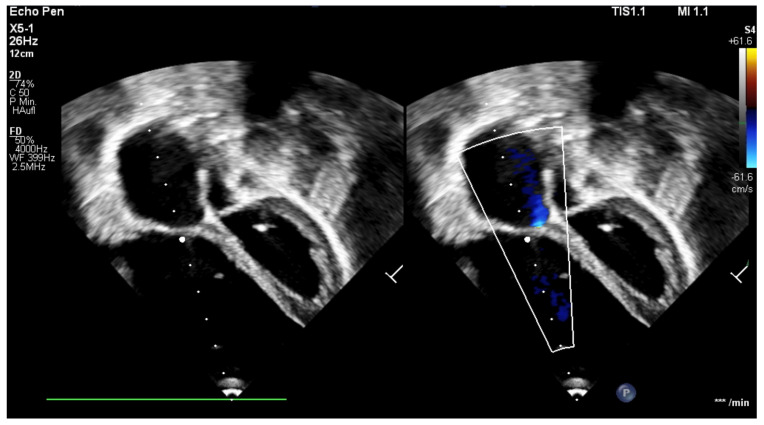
Echocardiography, four-chamber view; 18 months after cone reconstruction, 1 week after cardiac catheterization with stent implantation in the right pulmonary artery. * = heart beats/minute, heart beats were not derived in this echocardiography.
